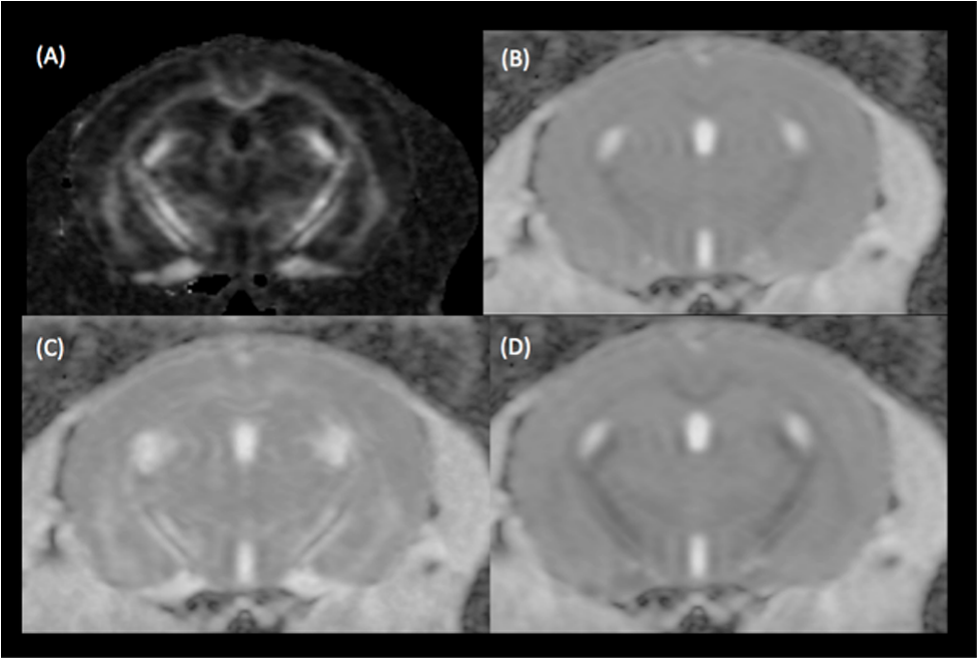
Examples of the DTI derived parameters of the level of the mid-brain structures from *in vivo* 2D EPI DWI at 16.4 T. (A) FA, (B) MD, (C) AD and (D) RD maps.
